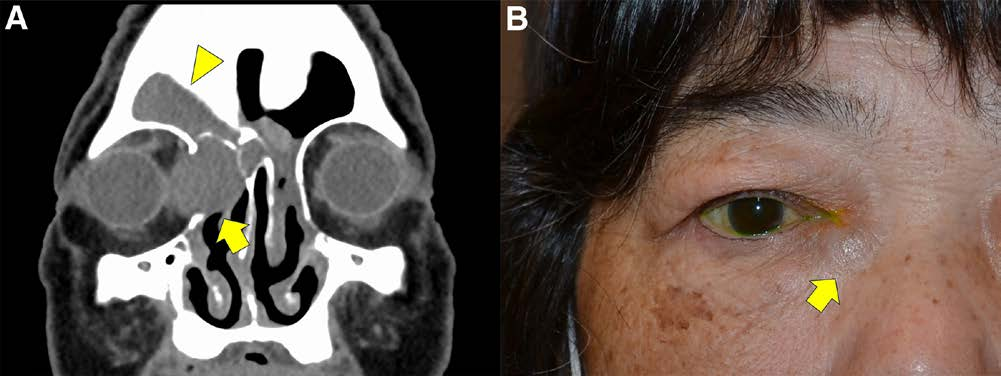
Clinical images. (A) A coronal computed tomographic image in Case #1 showing both lacrimal sac enlargement (arrow) and frontal sinus mucocele (arrow head). (B) A face photo in Case #2 showing a bluish lesion in the medial canthus (arrow).
